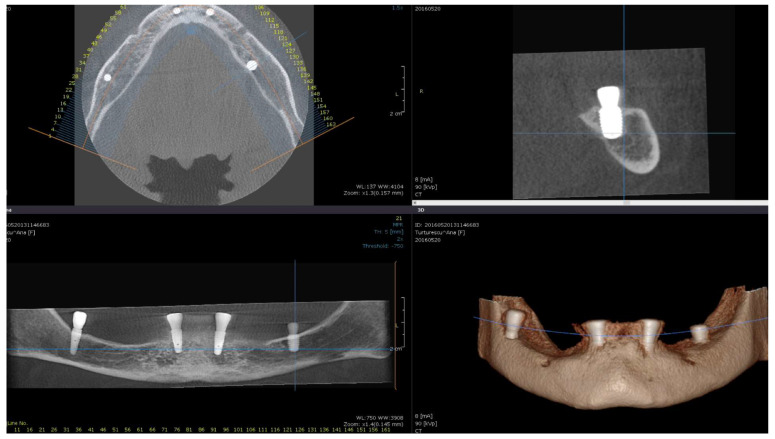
Postoperative CBCT of patient no. 5: (Upper left)—axial view of the mandible; (upper right)—sagittal left view of the lingually tilted implant (36); (lower left)—coronal view of the mandible; (lower right)—3D view of the mandible.
